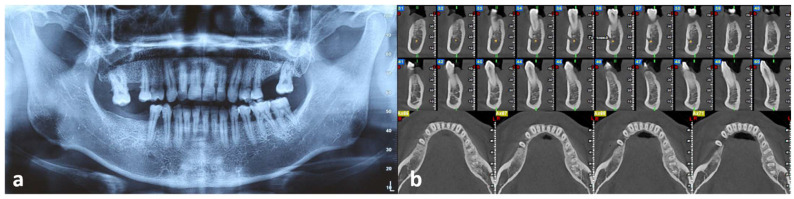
(a) Panoramic radiograph showing alveolar bone loss in proximity to the right mandibular premolar area. (b) Cross-sectional and axial views of cone-beam computed tomography (CBCT) revealing superficial resorption of the alveolar ridge and destruction of the buccal cortical bone in the area of the right mandibular premolars.
